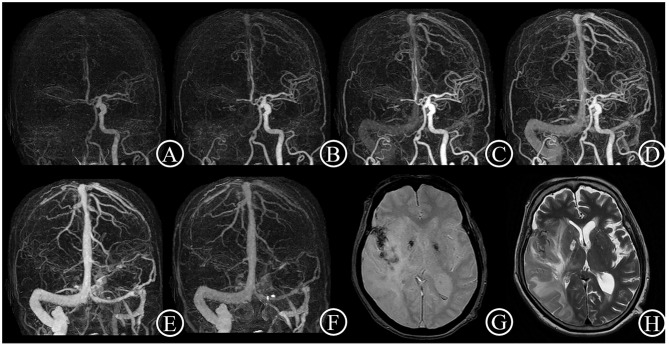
Poor collateral case. One 84-year-old female patient suddenly suffered from inability to lift left upper limb for 1.5 h. She had an initial NIHSS score of 11 and a mRS score of 4 after 3 months. 4D CTA (A–F) showed occlusion of right distal carotid artery and right middle cerebral artery, and few collateral vessels in the lesion side in any phase. Thus, the patient got a 4D CTA collateral circulation score of 0. The T2 star (G) and T2WI (H) on day 7 showed a large acute infarct lesion with hemorrhage transformation.
